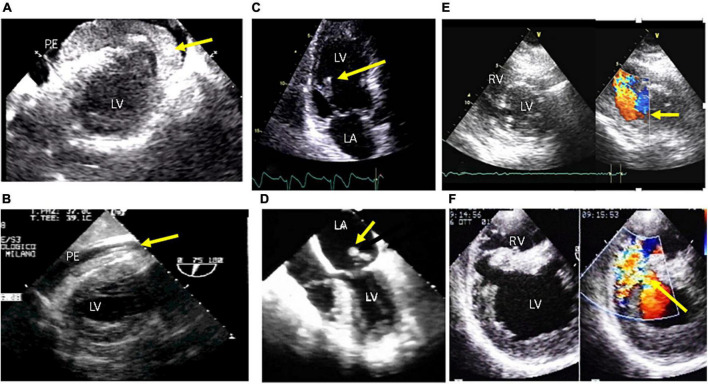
Echocardiographic aspects of mechanical complications of acute myocardial infarction. Upper panel: transthoracic echo. Lower panel: transesophageal echo. LV, left ventricle; RV, right ventricle. (A,B) Free wall rupture with pericardial effusion (PE) and thrombosis intrapericardial hematoma in the pericardial space (yellow arrows); (C) partial papillary muscle rupture (yellow arrow); (D) Complete papillary muscle rupture (yellow arrow) with eversion in the left atrium (LA); (E,F) ventricular septal defect with left-to-right shunt across the septum (yellow arrows).
